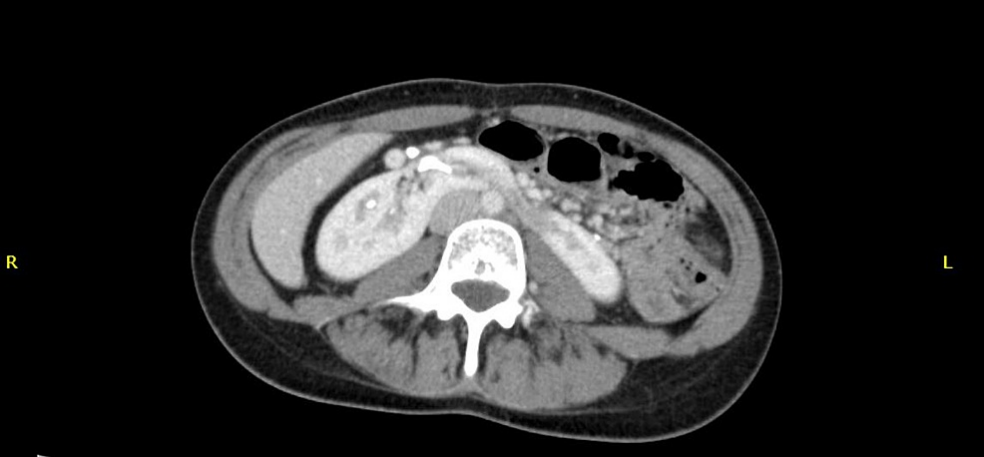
Abdominal CT scan without contrast shows resolution of abscesses.
